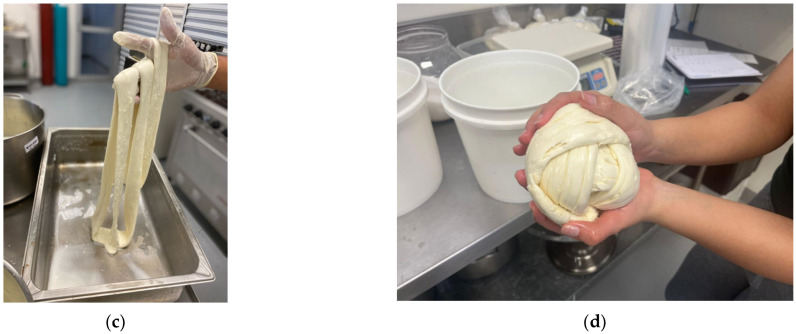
Images of Oaxaca cheese showing differences in appearance (a) and in the formation of strings or “threads” (b) due to the effect of ultrasound treatment (0, 15, and 30 min of HIU, 25 or 45 kHz, left to right and top to bottom); stretched (c) and ball forming or molded (d).
